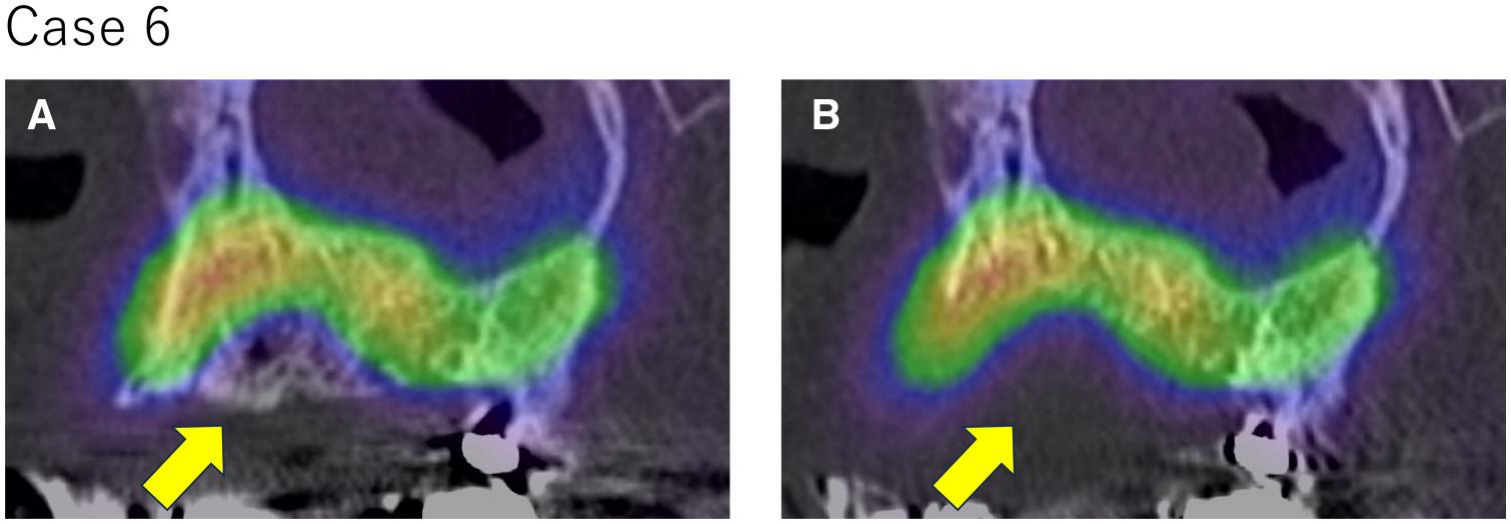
Fusion images of (A) pre or (B) postoperative CT and preoperative bone SPECT for Case 6. Yellow arrows indicate the resection region. The resected area included the cold region observed in bone SPECT and radiotracer accumulation was confirmed around the resected area.
